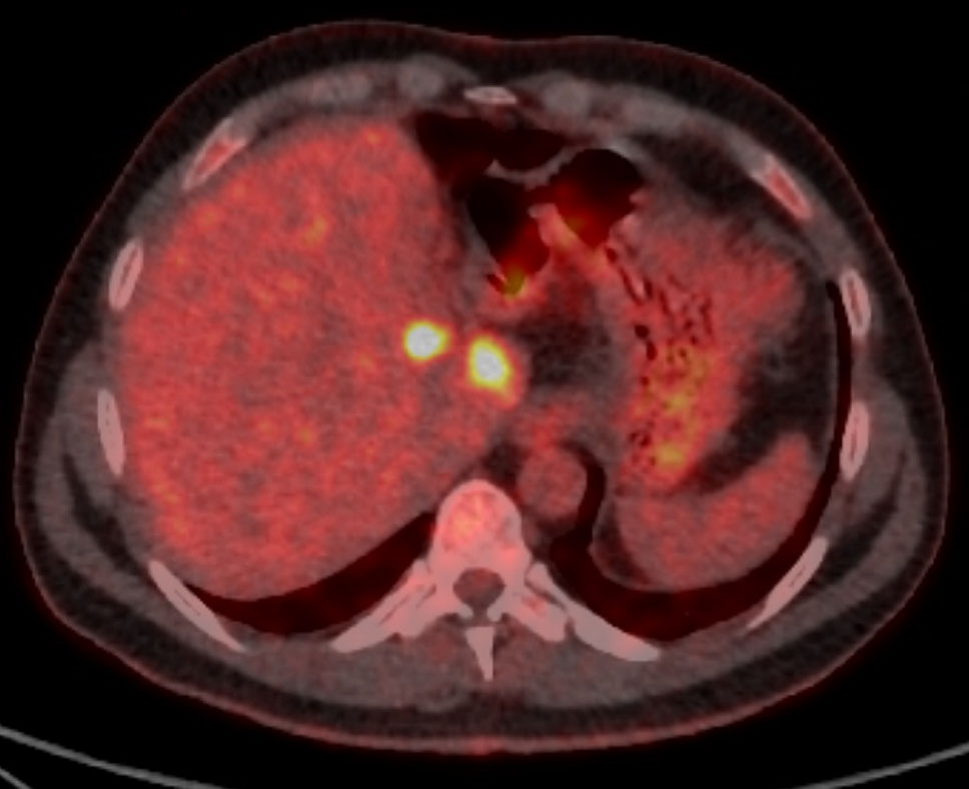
48-year-old male with colorectal liver metastases. Surveillance PET-CT imaging detected recurrence adjacent to the resection margin and either side of the left portal vein unsuitable for thermal ablation
